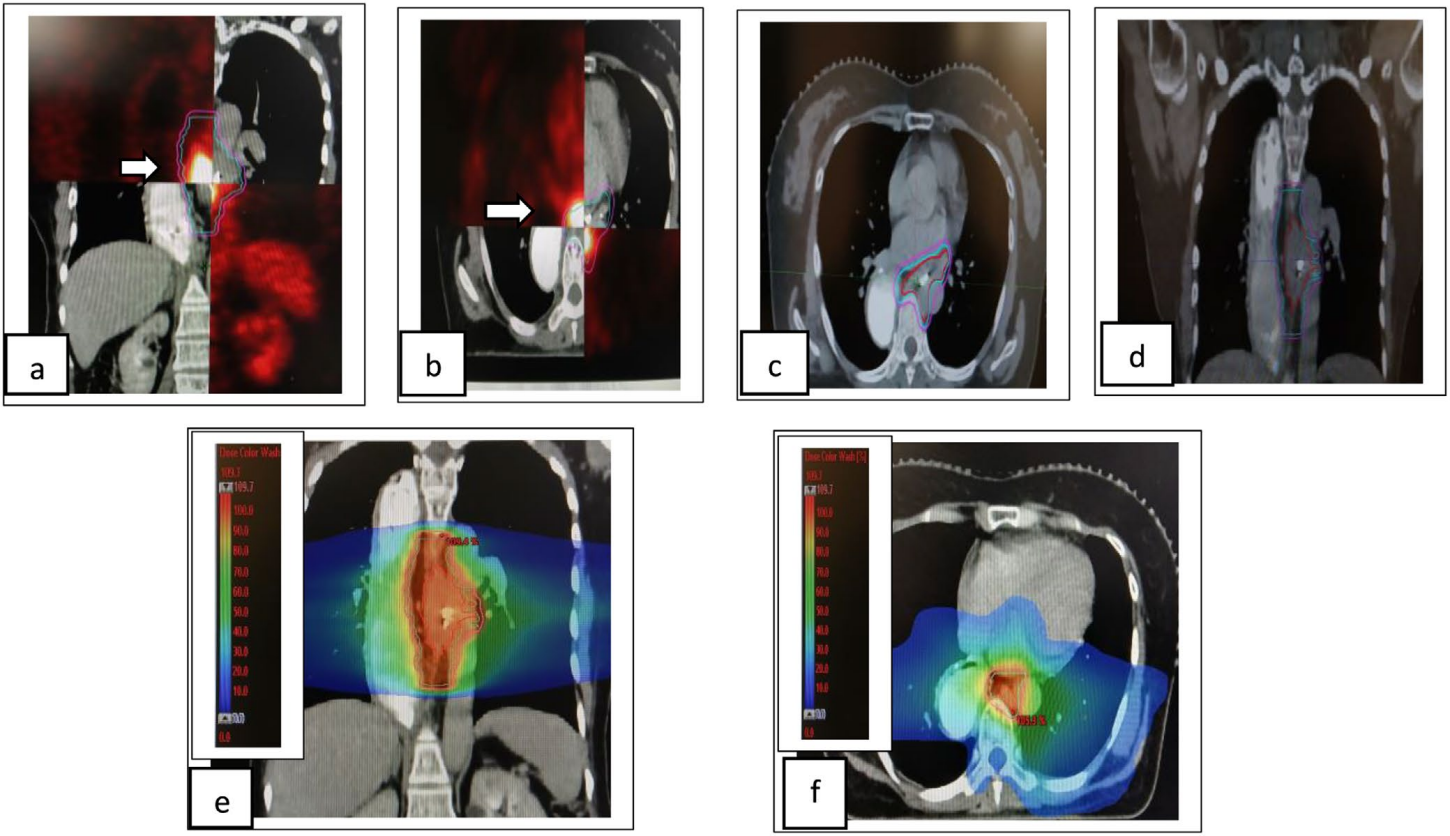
Second recurrence in the mediastinal region. (a, b) White arrow in coronal and axial sections of CT superimposed with PET shows second mediastinal nodal recurrence. (c, d) Axial and coronal CT section shows GTV (red), CTV (sky blue), and PTV (pink) for the second mediastinal recurrence. (e, f) Coronal and axial CT section shows dose color wash.
